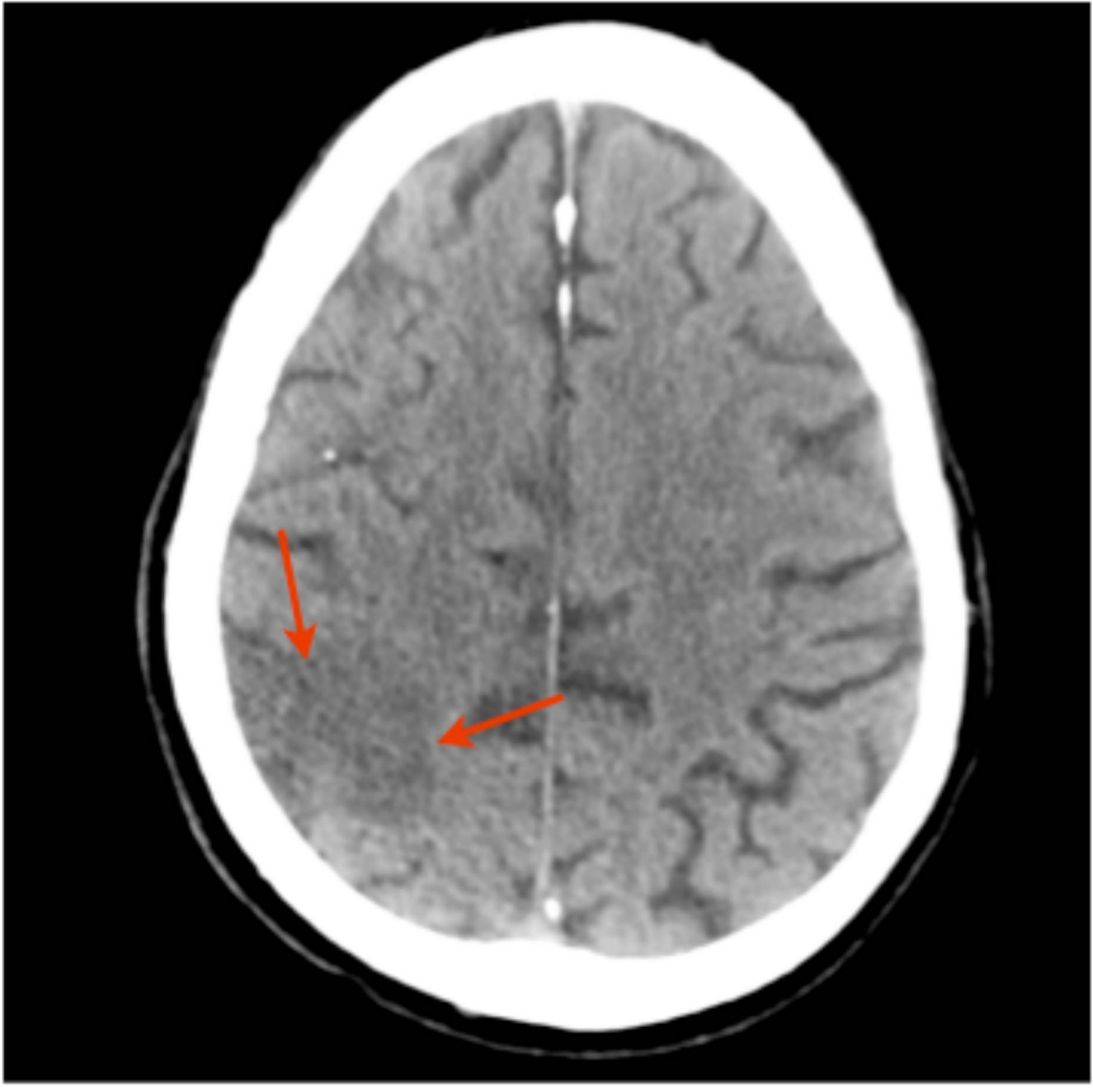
CT scan of the brain. Scan taken 48 hours post-admission. The report stated a right-sided hypodense area that was most likely to be a right-sided parietal infarct. CT: computed tomography
